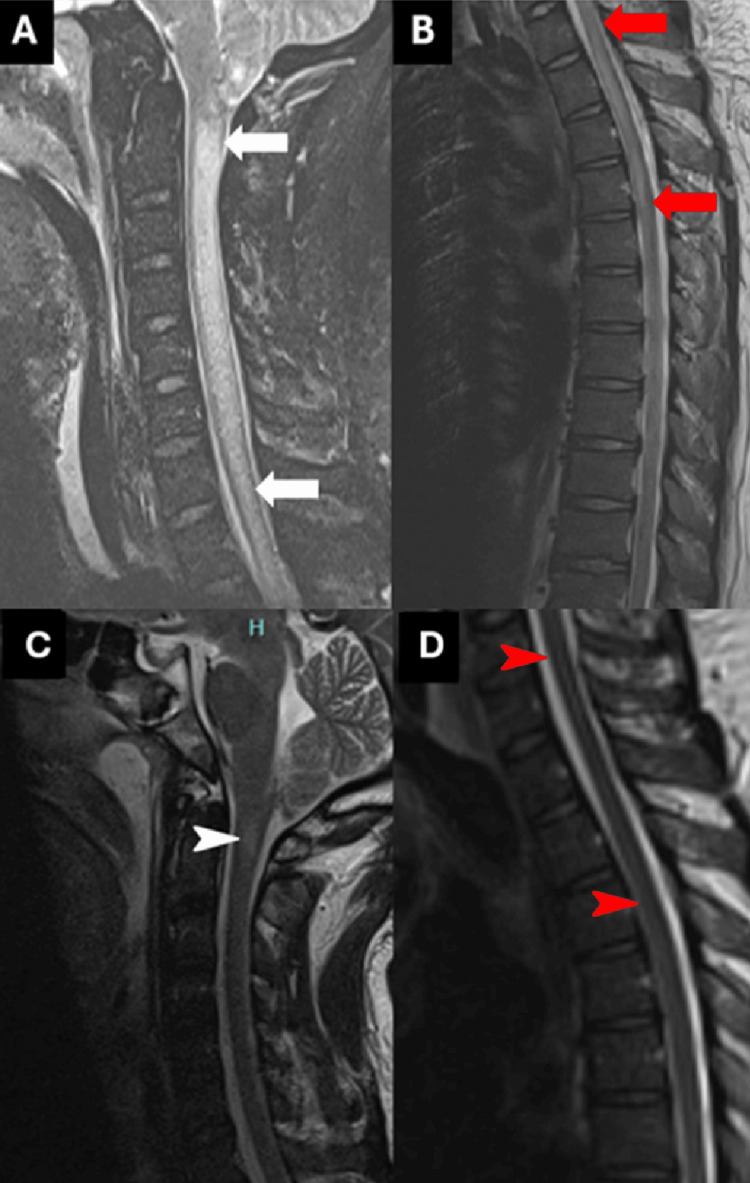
Initial sagittal T2 sequence of cervical and thoracic spine MRI showing multilevel intramedullary hyperintensity seen throughout the cervical spine (A, white arrows) and involving numerous segments of the upper thoracic spine (B, red arrows). Sagittal T2 sequence of cervical and thoracic spine MRI obtained three weeks after admission showing subtle hyperintensity at C1 level (C, white arrowhead), and resolution of intramedullary hyperintensity seen in upper thoracic spine (D, red arrowhead).
